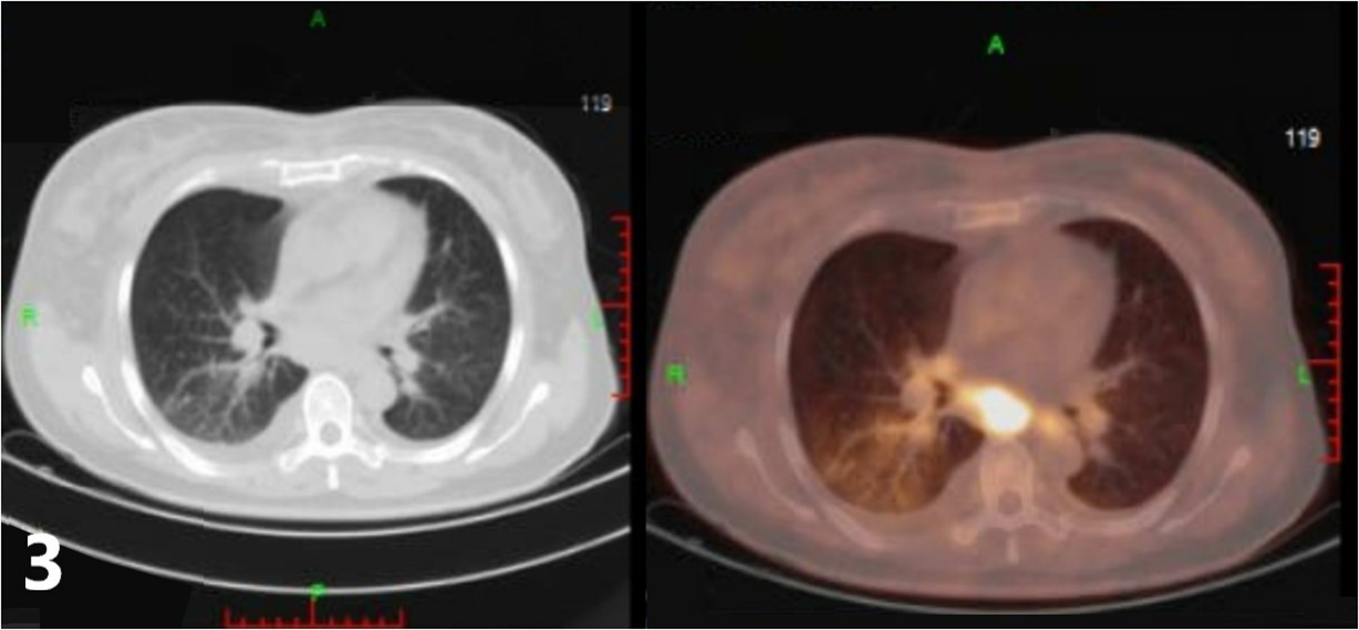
The18-fluorodeoxyglucose positron emission tomography-computed tomography scan (FDG PET/CT) of the lung revealed multiple bilateral pulmonary hypermetabolic foci.
